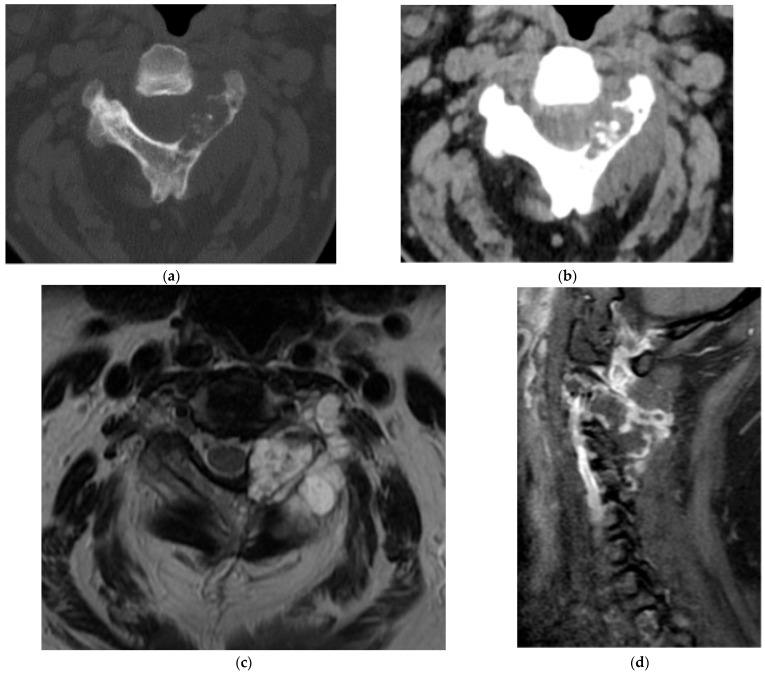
Chondrosarcoma. (a) Axial CT bone window, aggressive mass centered in the left lamina of C3 with chondroid matrix present centrally (rings and arcs). There is endosteal scalloping and cortical destruction. (b) Axial CT soft tissue window, extraosseous soft tissue component extending posteriorly from the left lamina mass. Chondroid matrix is again noted centrally. (c) Axial T2 MR: there is high T2 signal related to hyaline cartilage and increased water content. Mineralized “rings and arcs” are present centrally and low in signal. (d) Sagittal T1 fat saturation post-contrast, irregular peripheral enhancement.
